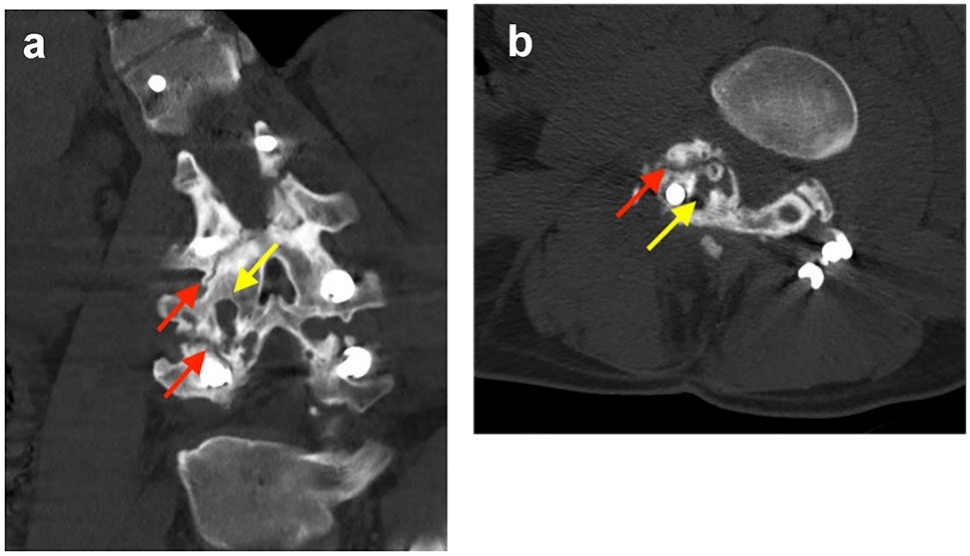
Coronal and axial computed tomography images showing pseudarthrosis (red arrow) and a bone cyst (yellow arrow)
